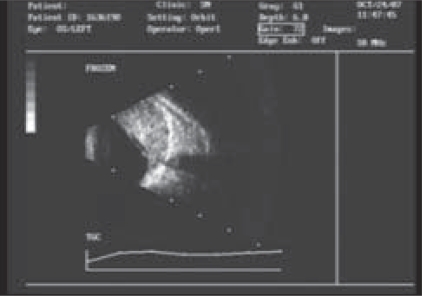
The ultrasonographic findings
